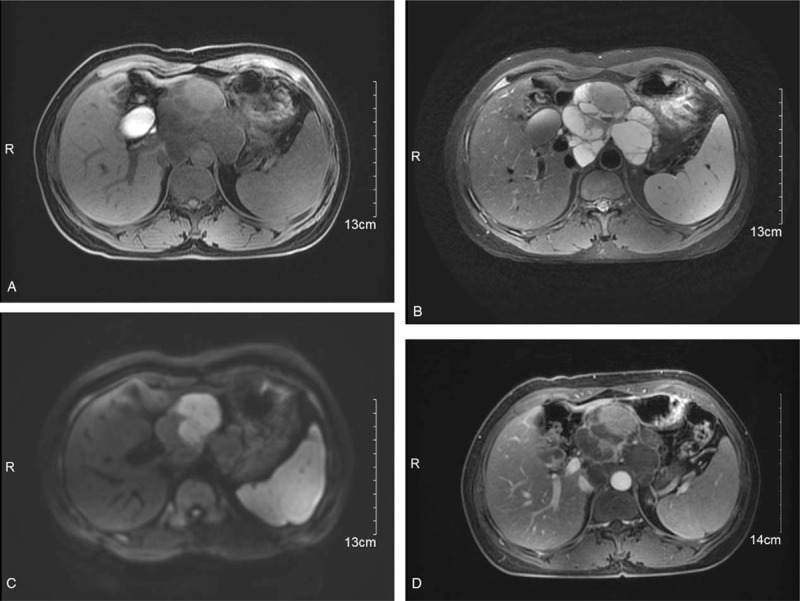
MR images showing a 9-cm × 8-cm cystic and solid mass in the retroperitoneum. (A) Axial fat-suppressed T1-weighted MR image showing a mass with heterogeneous hypointensity. (B) Axial T2-weighted MR image showing a mass with heterogeneous hyperintensity. (C) Diffusion-weighted image showing restricted diffusion in the solid portion. (D) Axial contrast-enhanced fat-saturated T1-weighted image showing marked and persistent enhancement of the solid portion and septations. MR = magnetic resonance.
